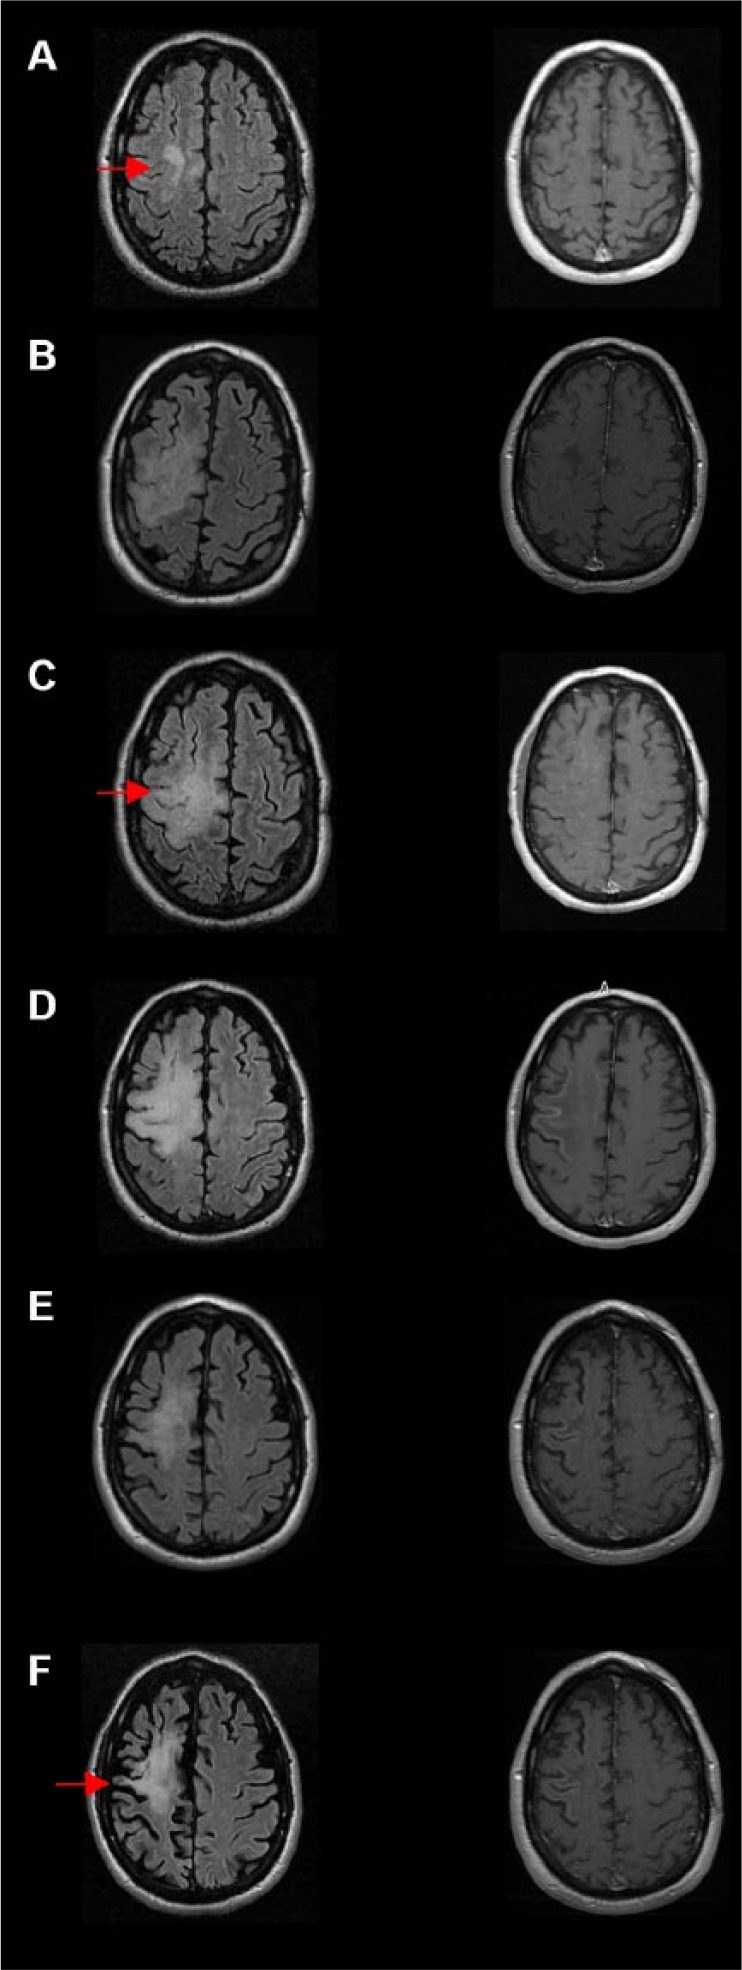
MRI progression of progressive multifocal leukoencephalopathy (PML) and immune reconstitution inflammatory syndrome (IRIS). (A) Initial imaging suspicious for PML. A new confluent hyperintensity was shown in the right superior frontal cortical and subcortical white matter region (arrow). This was confirmed to be PML with CSF JCV studies. (B) The patient presented 2 months later with worsened symptoms, and MRI showed worsened and enlargement of the lesion on T2 FLAIR (arrow; left column B, C, D), with patchy enhancement on T1 post contrast (right column B, C, D). The worsening clinical picture represents acute IRIS. Images C, D, and E show the progression of acute PML-IRIS lesions 2.5 months, 4 months, and 7 months from the diagnosis. (F) Decreased hyperintensity with increasingly apparent brain atrophy (arrow) was seen 14 months after the diagnosis. Left-side column: T2 FLAIR sequence; Right-side column: T1-post contrast.
